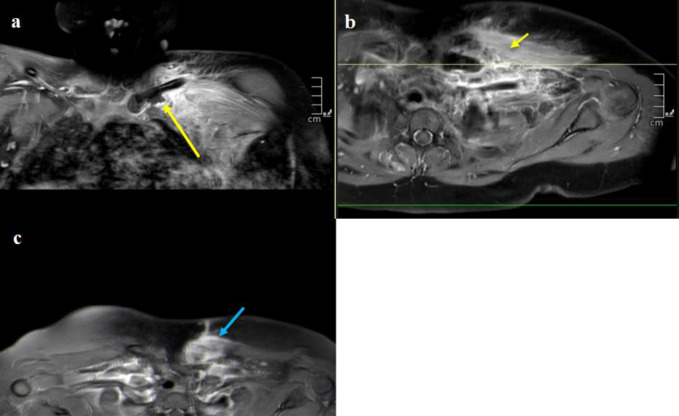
Patient 2 preoperative MR imaging. Coronal (A) and axial (B) T1 images after gadolinium administration demonstrating subperiosteal fluid collection (long arrow), likely due to metaphyseal extension from the epiphysis with surrounding edema in the chest wall musculature (short arrow), representing left SCJI. C, Subsequent T1 axial MR image with contrast 3 months after initial I&D demonstrating left medial clavicular osteomyelitis (blue arrow). I&D = irrigation and débridement, SCJI = sternoclavicular joint infection
